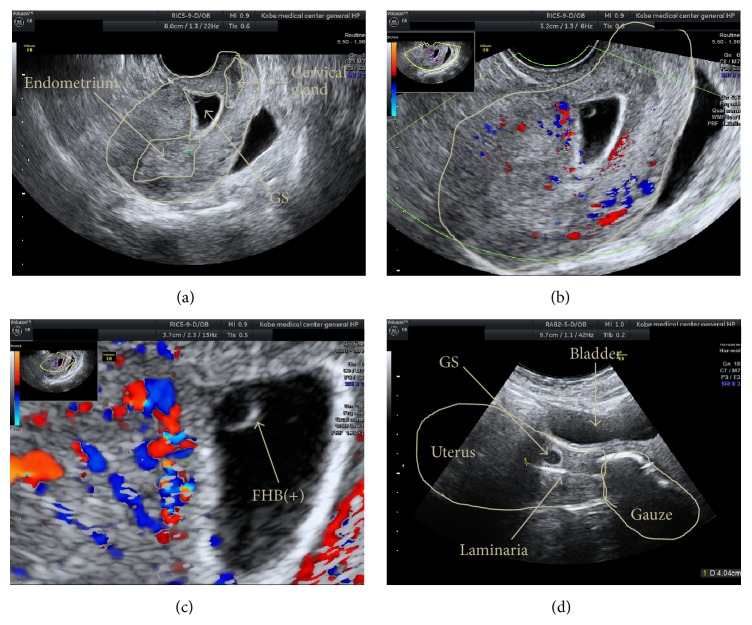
Ultrasonographic findings in cesarean scar pregnancy. (a) The gestational sac (GS) was located in the cesarean scar pouch. The uterine cavity and cervical glands were empty. (b) The GS was triangular in shape inside the cesarean scar pouch. (c) Fetal heartbeat was positive in the hyperechoic portion of the Yolk Sac, and the fundal side of the triangular-shaped GS was rich in blood supply. (d) The GS shape changed to round after insertion of the Laminaria tent.
